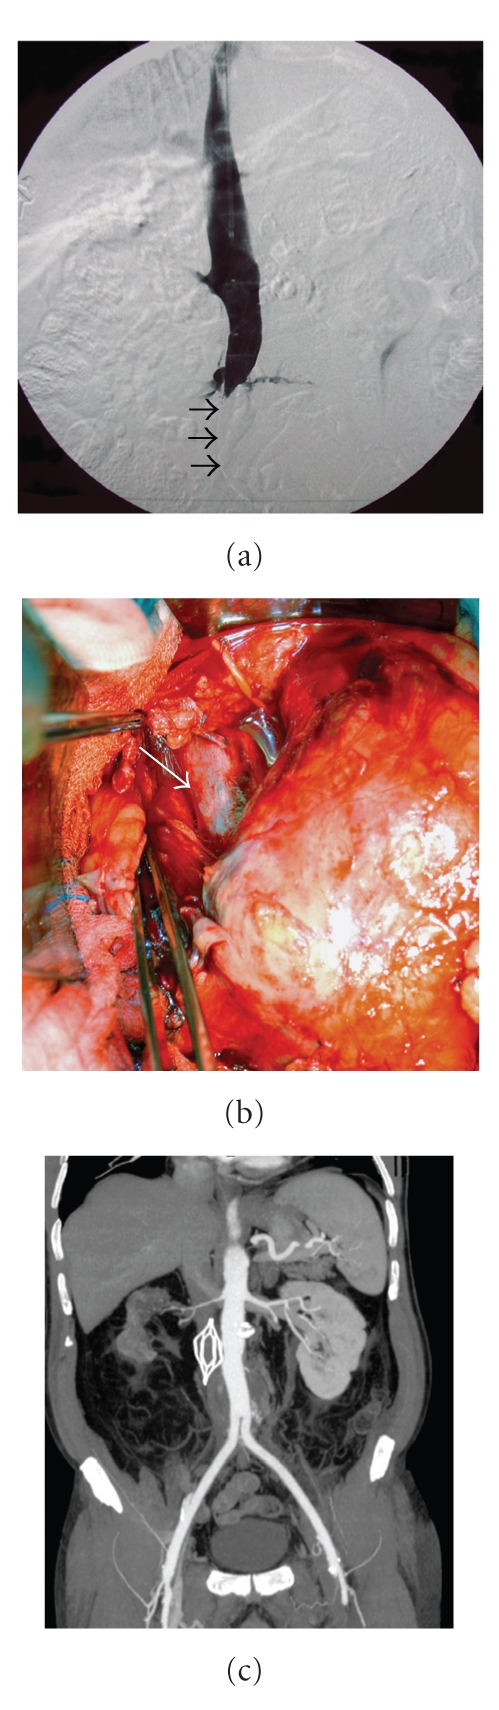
(a) Inferior vena cavagram performed through jugular approach demonstrates collapse of IVC at the AAA level (Black arrows). (b) Operative photograph shows IVC (white arrow) compressed medially and anteriorly by AAA. (c) Eight-month-postoperative coronal CT reconstruction shows patency of the vascular graft and of the IVC at filter level and cranially.
